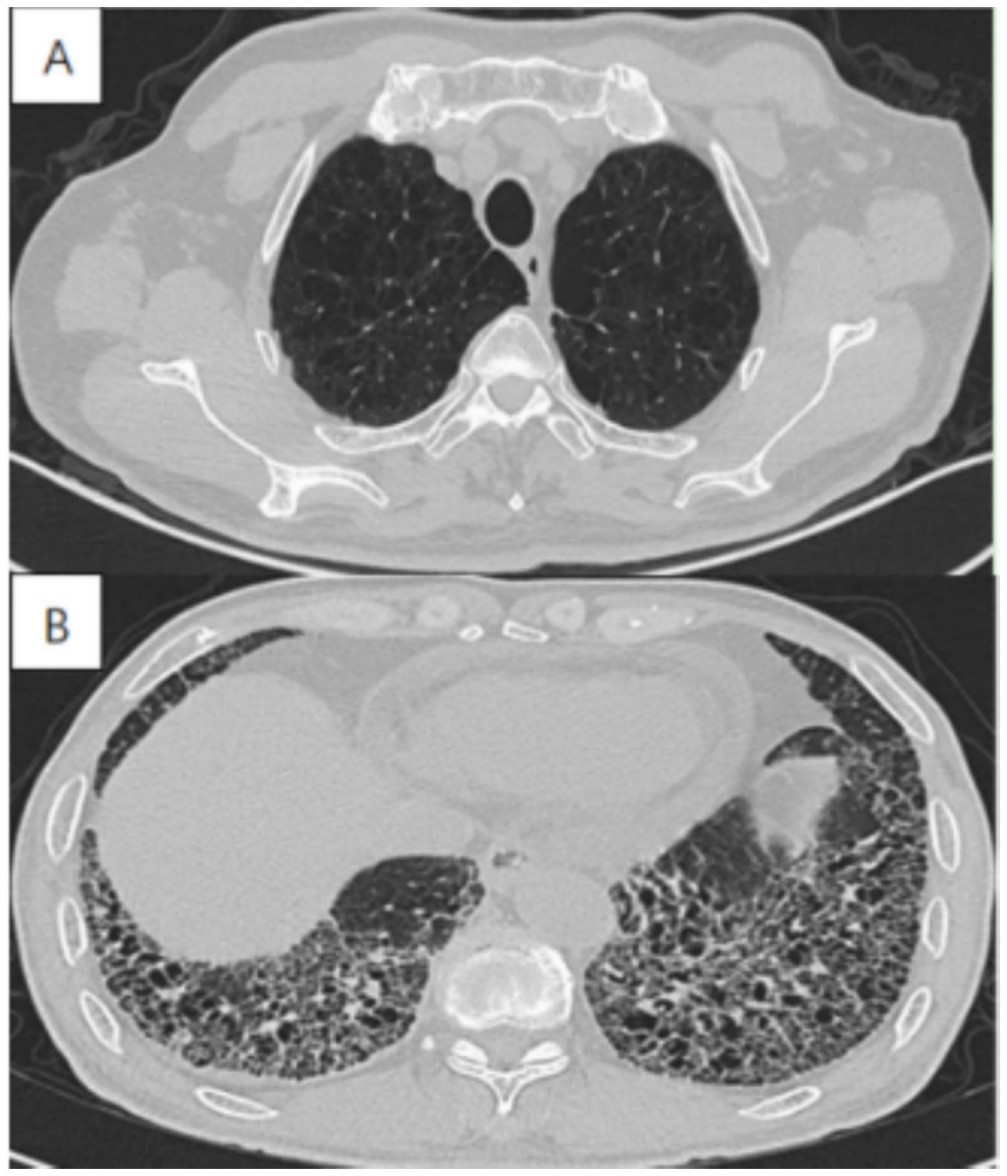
High-resolution computed tomography in a 72-year-old male with CPFE, showing (A) paraseptal emphysema in the upper lobe, (B) lower-zone-predominant fibrosis.
